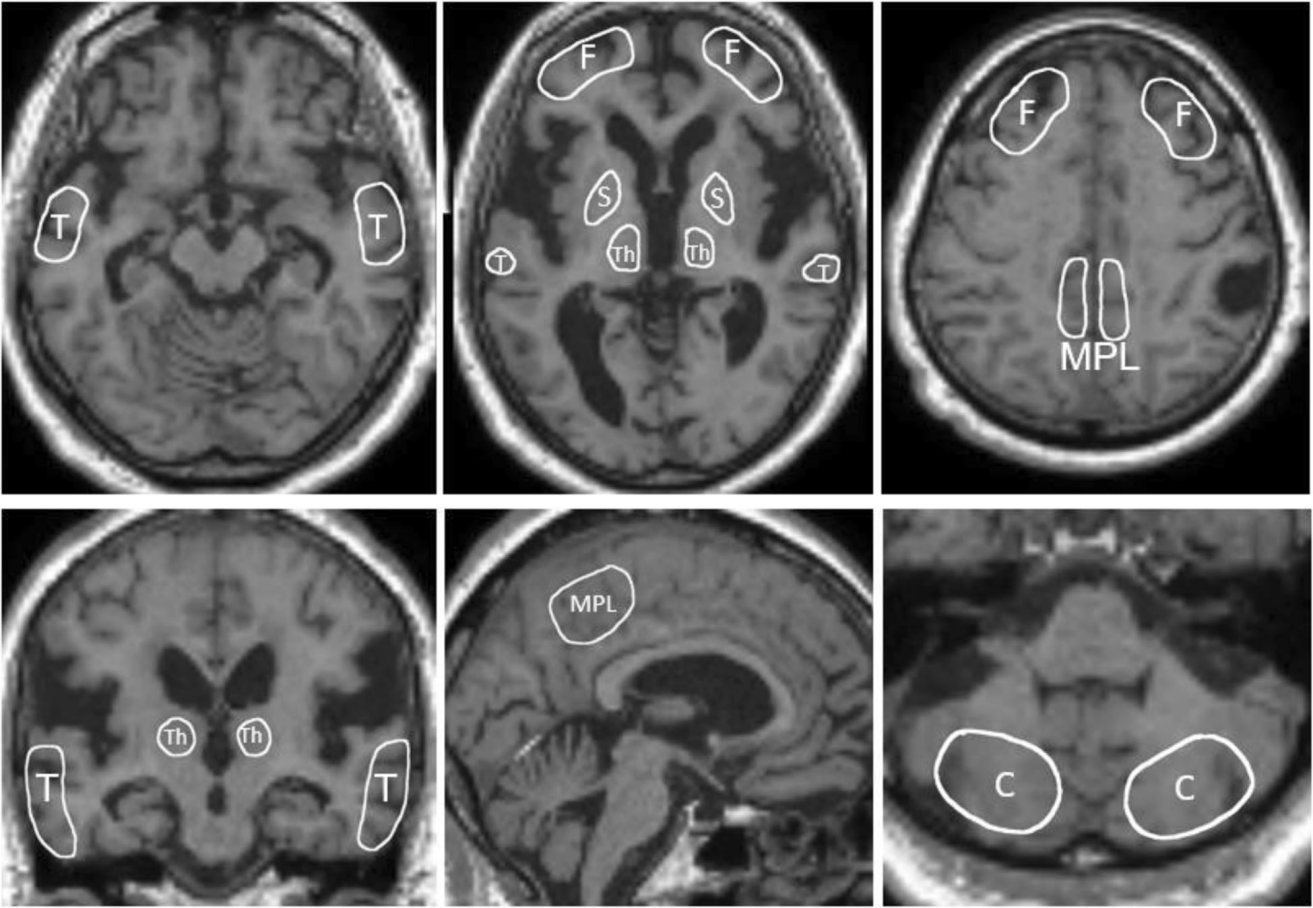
Representative slices of volumes of interest (VOIs) used to evaluate lesion-to-cerebellum standardized uptake value ratio (SUVRs). All VOIs were drawn to avoid areas of brain deformation, such as ESF, dilated ventricles, and focally dilated sulci. For the purpose of clearly showing the location of the VOI, this figure was created using magnetic resonance imaging (MRI) images of DESH. *F* frontal lobes, *T* temporal lobes, *MPL* medial parietal lobes, *S* striata, *Th* thalami, *C* cerebellum
